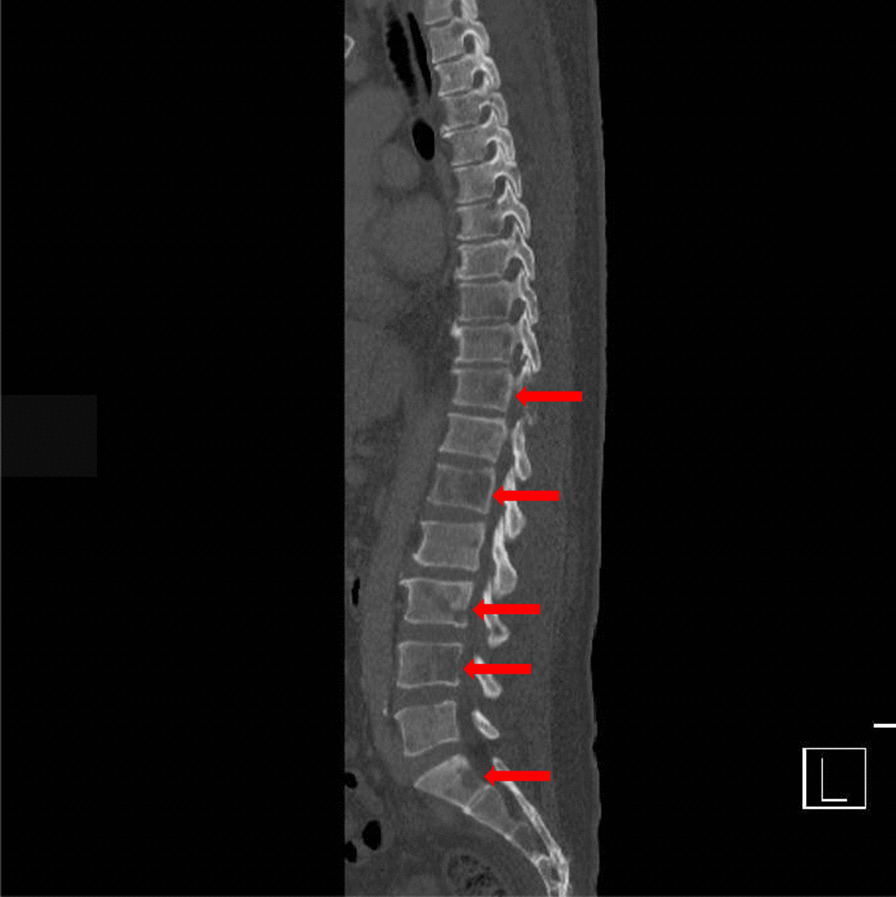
Computed tomography image showing multiple osteolytic lesions throughout the spine (red arrows)
